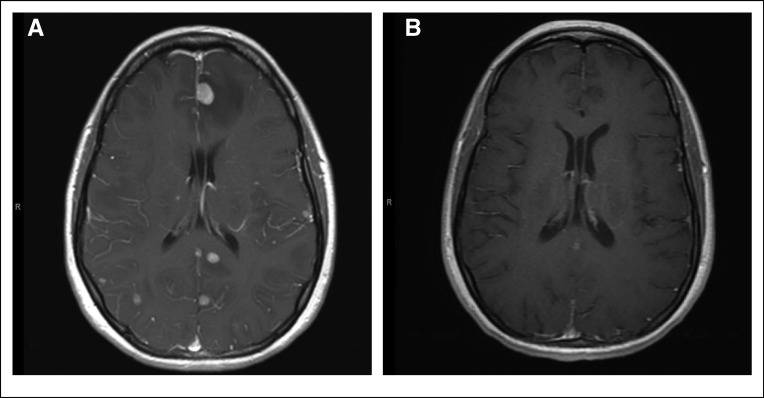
Magnetic resonance images of the brain (T1 axial postcontrast series) (A) before and (B) 1 month after treatment with lorlatinib, showing significant response of all metastatic brain lesions.
